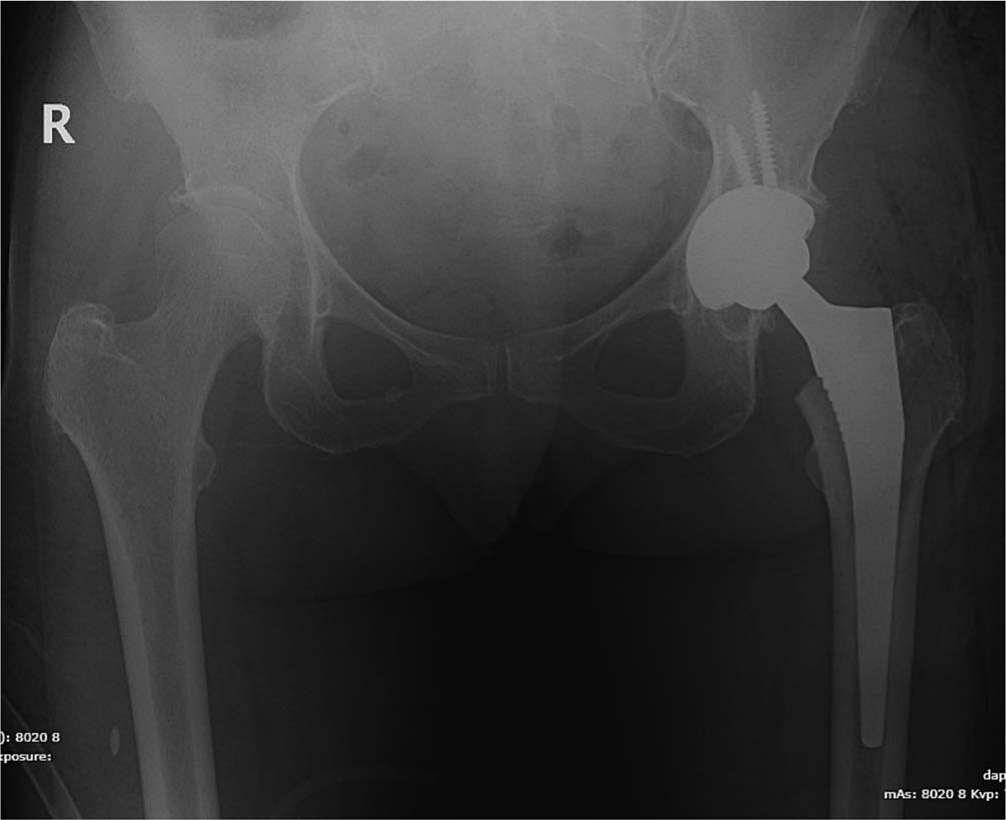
X-ray pelvis showing left total hip replacement with hardware in adequate position.
